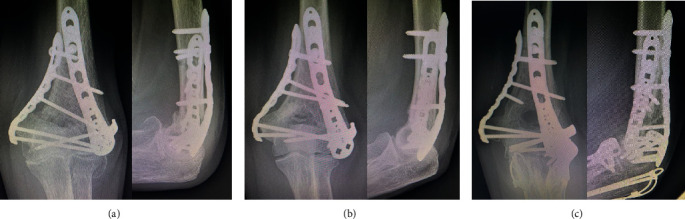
X-ray images at the final follow-up: (a) patient 1; (b) patient 2; (c) patient 3.
